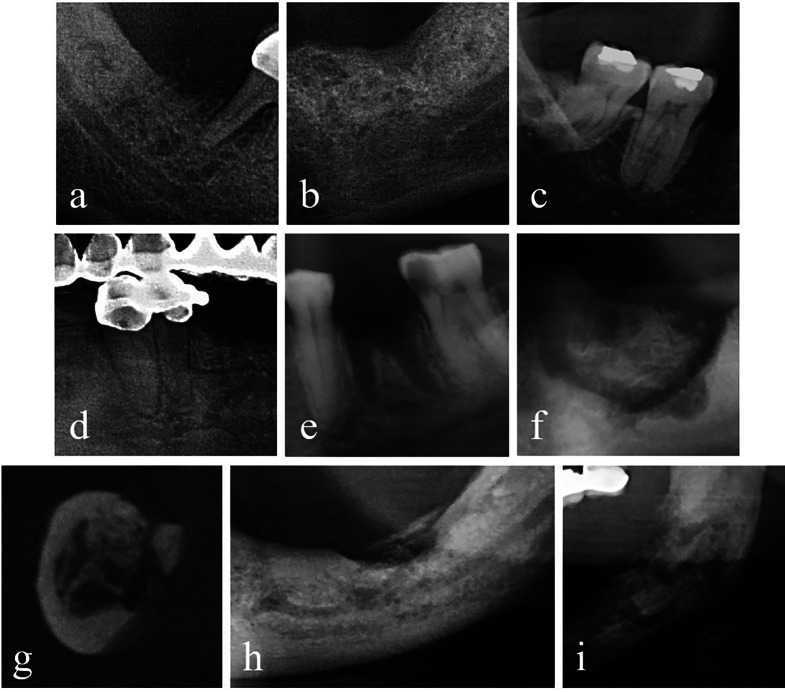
Representative PR and CBCT images demonstrating qualitative radiographic parameters. Cropped PR images showing (a) osteolysis, (b) osteosclerosis, (c) LD thickening, (d) PDL widening, (e) persistent extraction socket, (f) sequestration. (g) Cropped coronal CBCT image showing periosteal new bone formation. Cropped PR images demonstrating (h) narrowing of the inferior alveolar canal and (i) pathologic fracture
